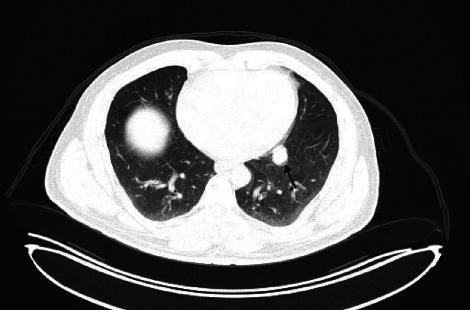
High-resolution chest CT showing another left lower lung lobe paracardiac lung mass denoting another metastatic lesion.
